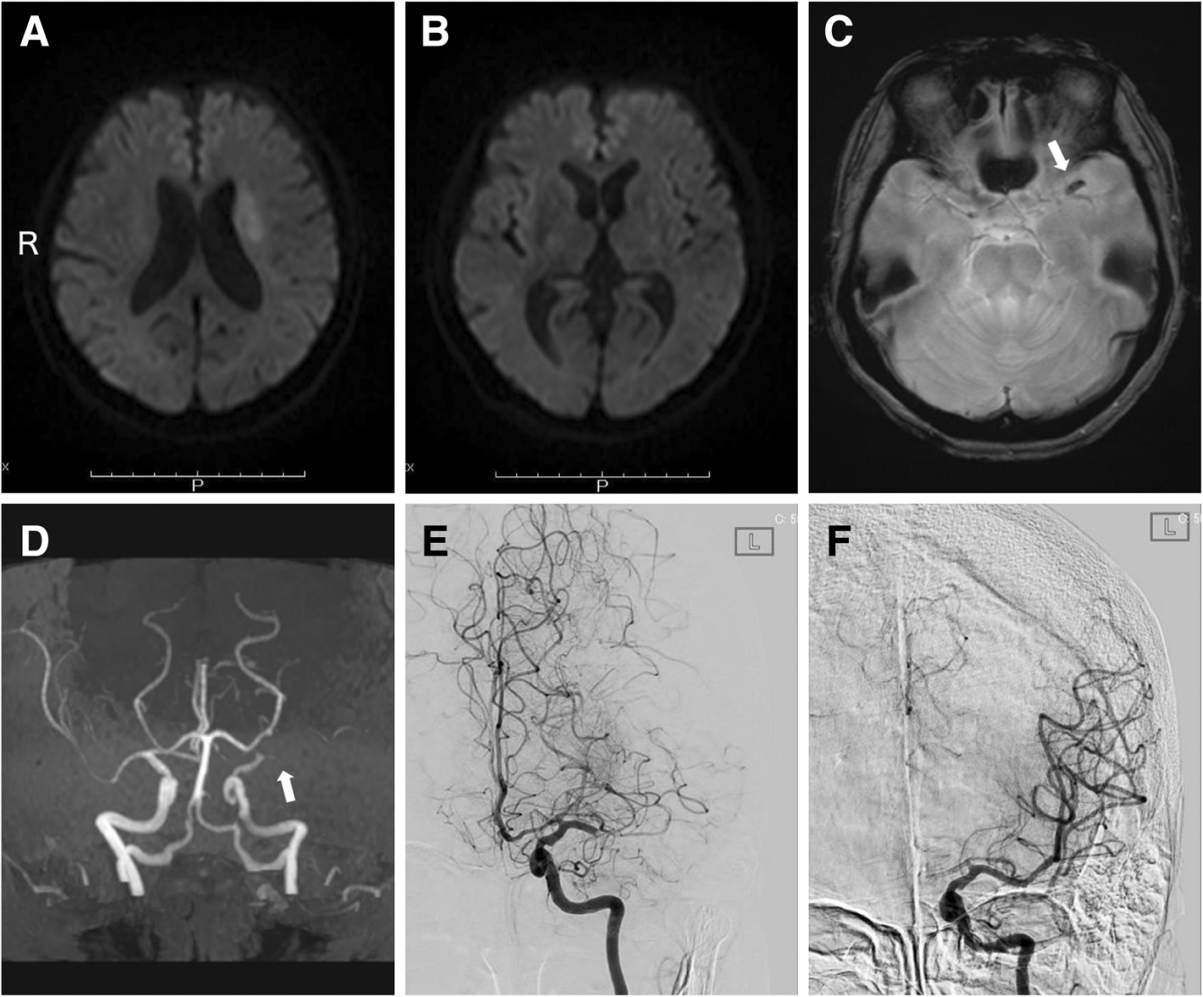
Diffusion-weighted magnetic resonance imaging showing acute infarction at the left corona radiata (a) and left basal ganglia (b). (c) T2*-weighted imaging showing a susceptibility vessel sign (white arrow) in the left middle cerebral artery. (d) Magnetic resonance angiography showing proximal occlusion (white arrow) of the left middle cerebral artery. (e) Anteroposterior view of a selective left internal carotid angiogram showing occlusion of the left middle cerebral artery. (f) Left internal carotid angiogram, anteroposterior view, showing complete recanalization of the left middle cerebral artery
